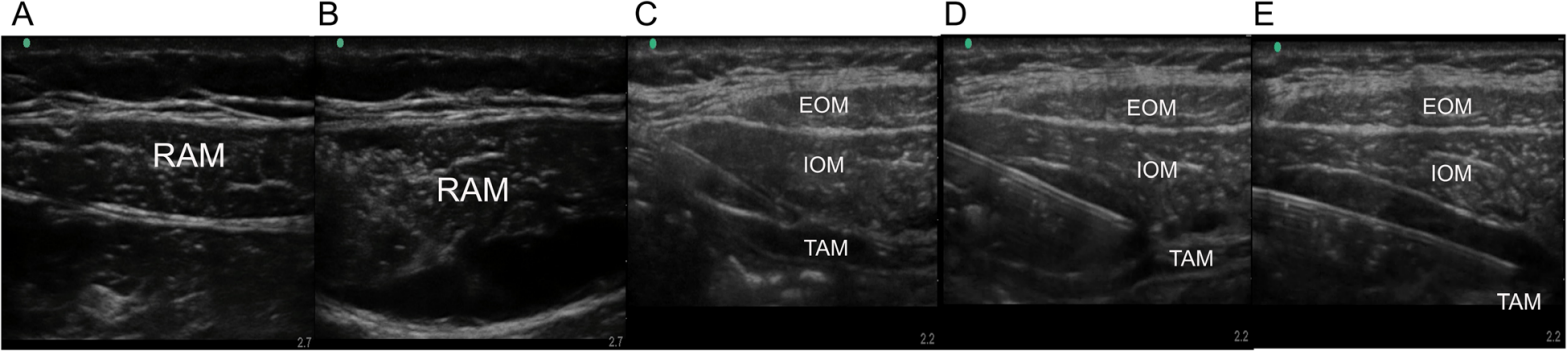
Ultrasound-guided TAPB and RSB External oblique muscle, internal oblique muscle, transversus abdominis muscle, and peritoneum. A and B show ultrasound-guided RSB. The needle tip was positioned in the posterior rectus sheath, and saline was injected. Uniform hydrodissection of the muscle tissue and sheath was critical for the success of RSB, and then, the anaesthetics were injected. *RSB* rectus sheath block, *RAM* rectus abdominis muscle. C, D and E represent ultrasound images of TAPB. The needle tip was positioned in the plane between the internal oblique muscle and the transversus abdominis muscle. After dissection of the plane by injection of saline, the anaesthetics were injected. During the injection of anaesthetics, the needle was advanced further along the transabdominal plane, and the regional anaesthetics were injected step by step to ensure that the entire transabdominal plane was filled with anaesthetics. *TAPB* transversus abdominis plane block, *EOM* external oblique muscle, *IOM* internal oblique muscle, *TAM* transversus abdominis muscle.
